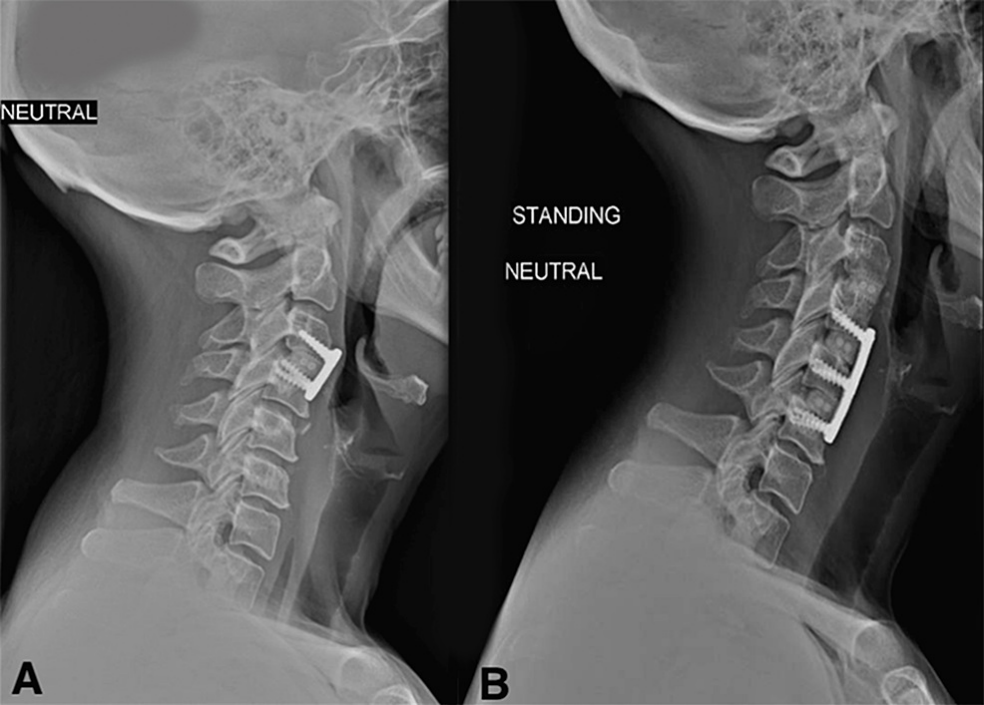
Anteroposterior radiograph of a case presentation of revision ACDF using traditional interbody PEEK cage, titanium plate, and screw instrumentation ACDF, anterior cervical decompression and fusion; PEEK, polyetheretherketone
